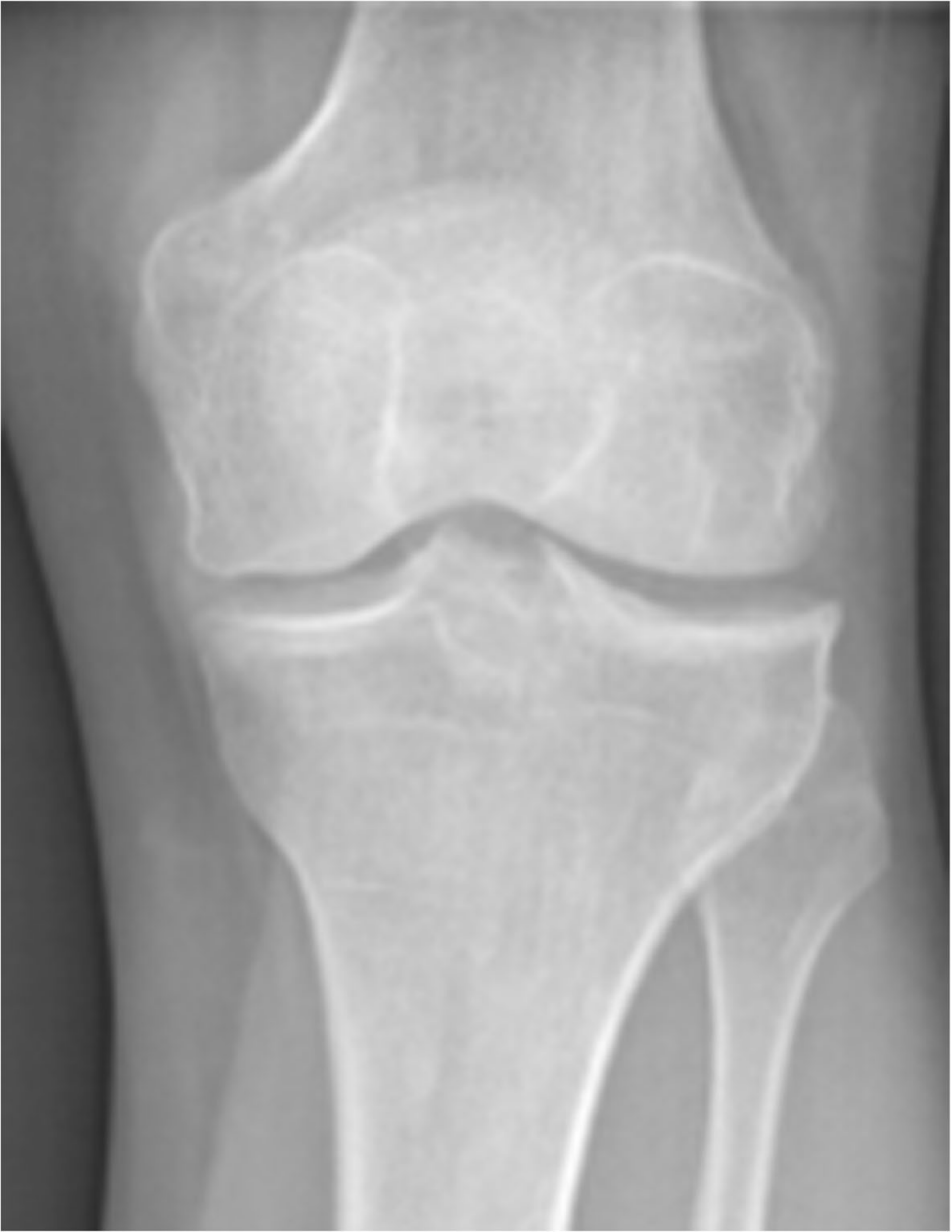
Pre-operative AP knee radiography Patient 2
